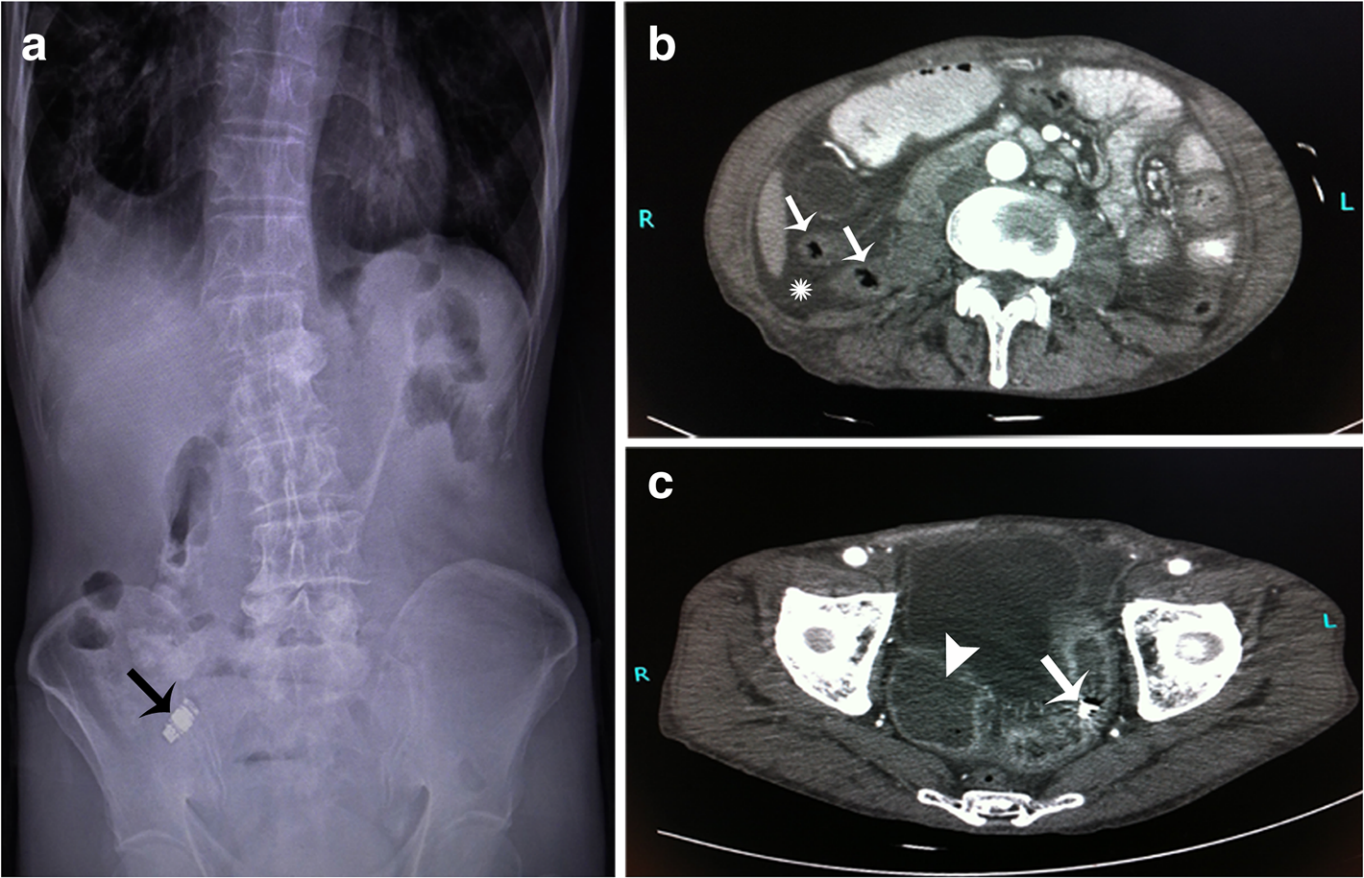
Radiographic examination of the abdomen. (a) Abdominal radiograph showed air-fluid levels and the endoscopy capsule (arrow) in the right lower quadrant. Oral contrast computed tomography showed (b) intestinal edema (arrows) and ascites (*) and (c) a high density object in the enteric cavity (arrow) and proximal intestinal dilation (arrowhead).
